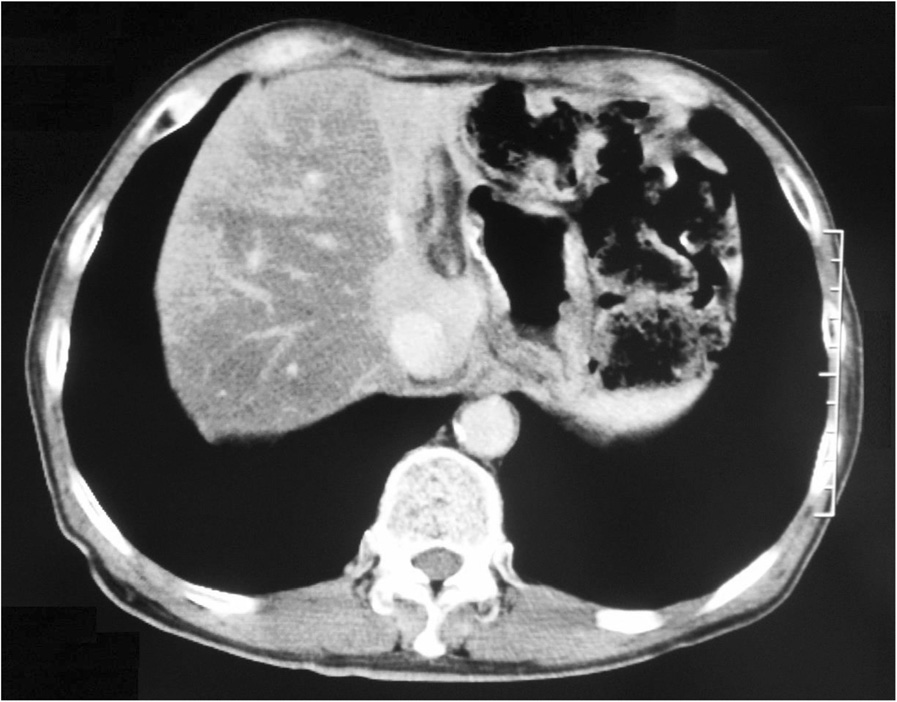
Abdominal computed tomography reveals that the tumor disappeared with atrophic change of the left lobe, together with a clinical complete response, 8 months after hepatic arterial infusion chemotherapy
